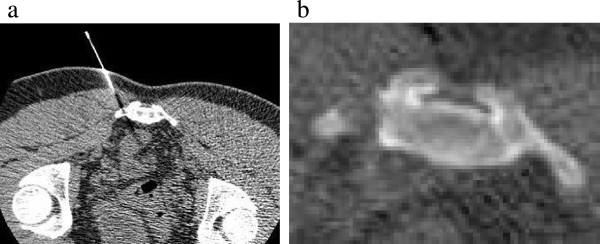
Computed tomography-guided resection. (a) A guide pin has been inserted into the nidus under computed tomography guidance. (b) Postoperative computed tomography shows a successful resection of the nidus.
